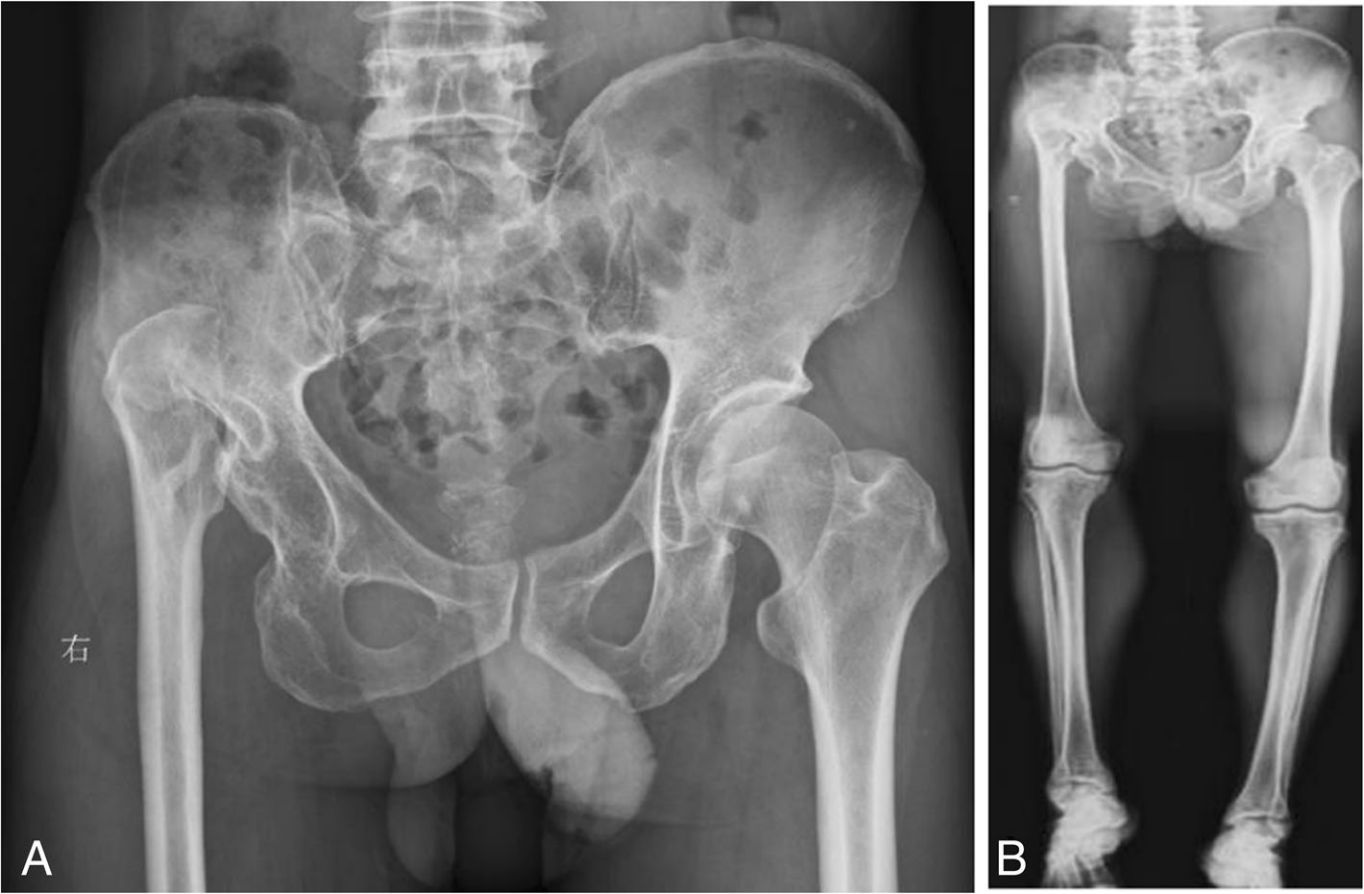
Radiographs of the pelvis and both lower extremities before the operation. (a) anteroposterior view of the pelvis, (b) anteroposterior view of both lower extremities
